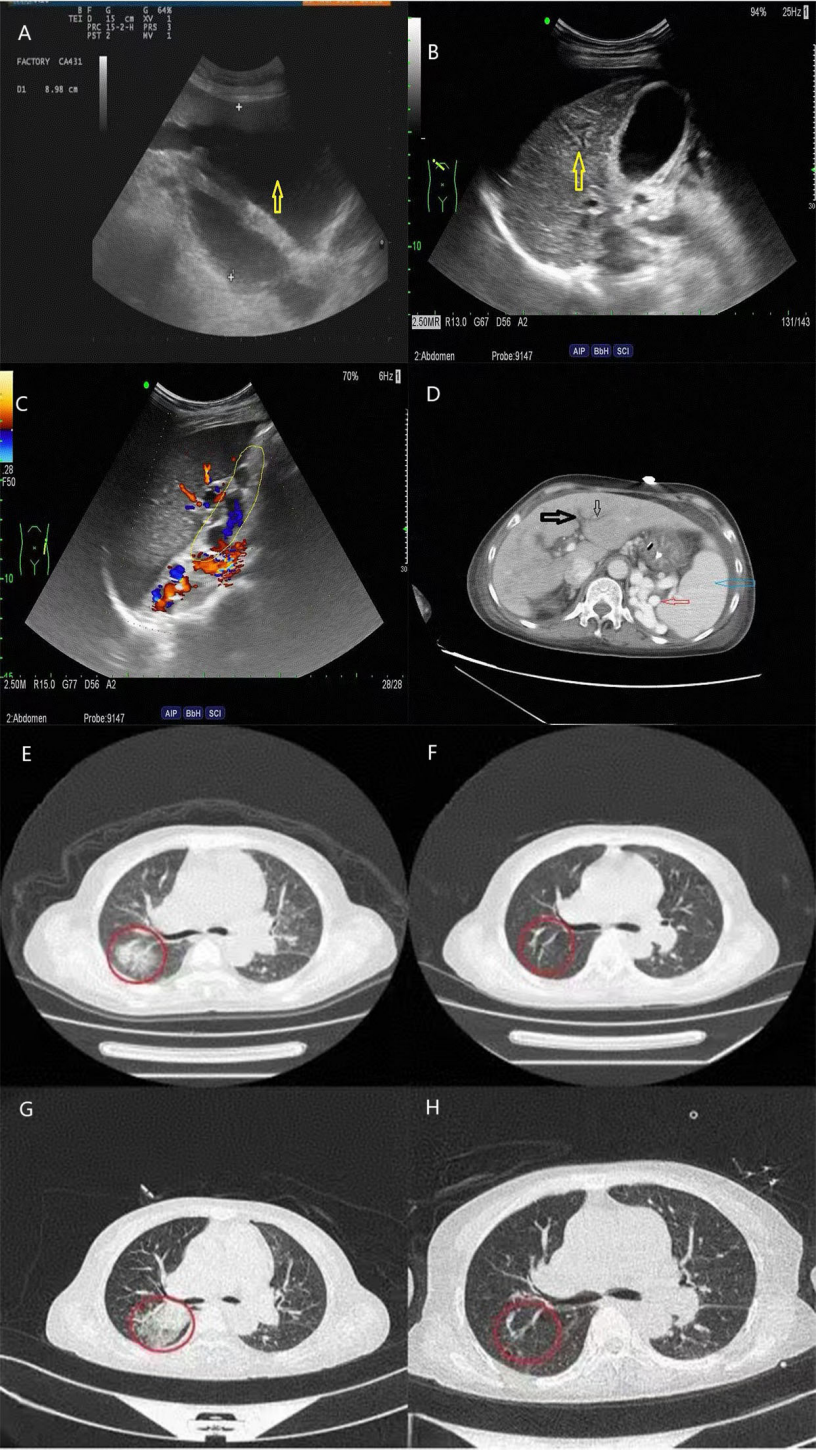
Abdominal ultrasonography and abdominal/chest CT scans of the patient. (A) Abdominal color ultrasonography indicates excessive ascites; (B) diffuse parenchymal thickening of the liver; (C) splenic veins are tortuous and dilated; (D) the black arrows show intrahepatic lymphatic dilatation; the blue arrow shows splenomegaly; and the red arrow shows collateral circulation; (E–H) show changes in chest CT infection lesions over time.
